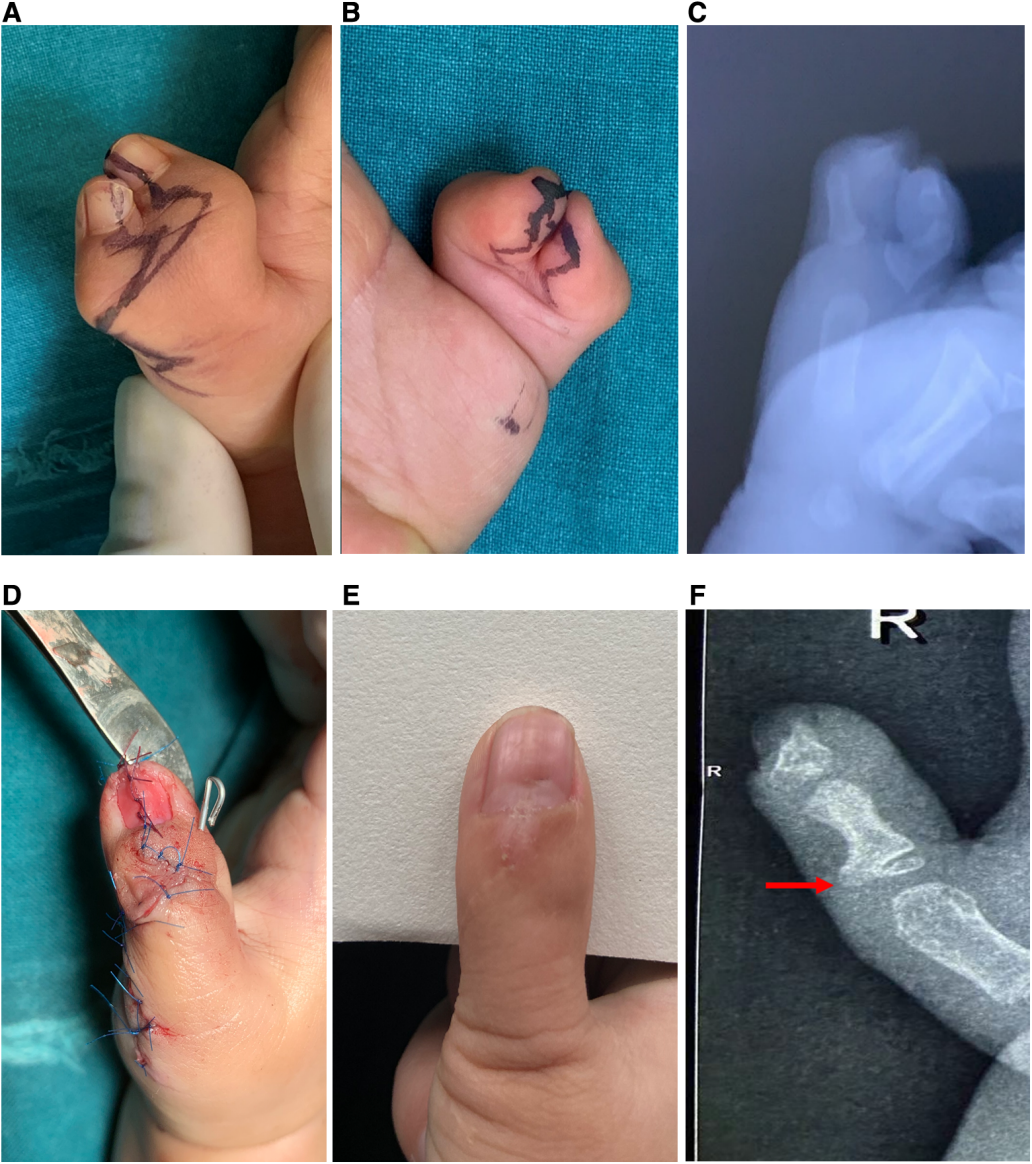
(A,B) Case 23, a lateral zig-zag incision; (C) preoperative x-ray; (D) postoperative appearance; (E,F) postoperative view, and postoperative x-ray after 15 months; red arrow shows radial protrusion of the proximal phalanx.
